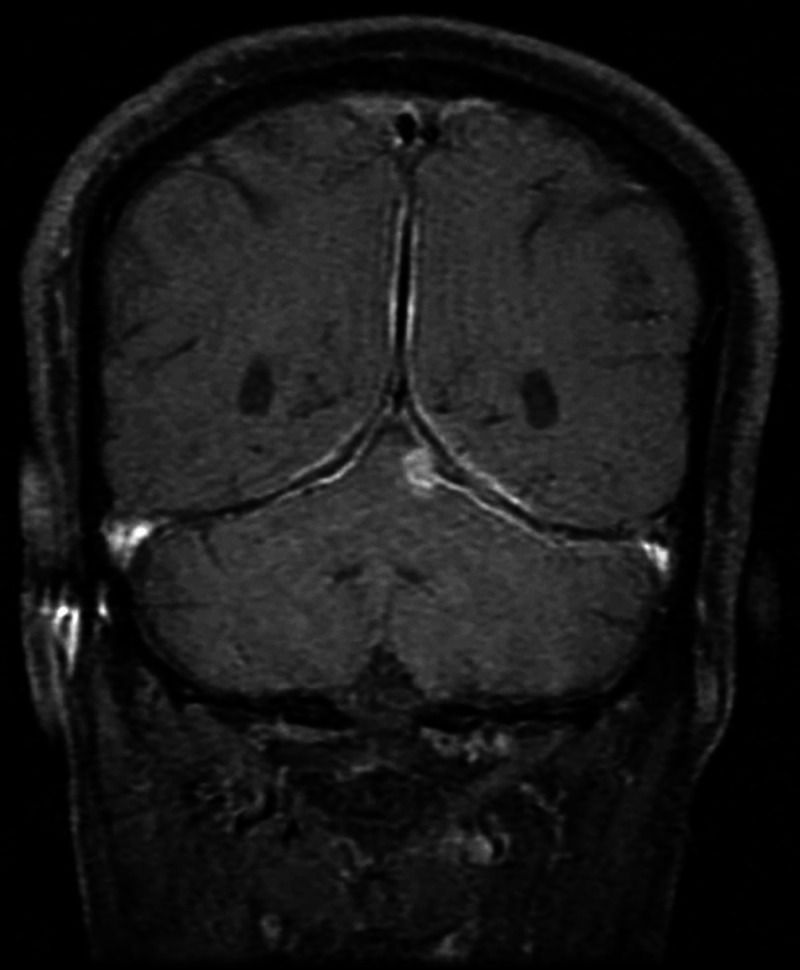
Posttreatment at 24 months: Post-gadolinium coronal T1-weighted MRI showing almost complete resolution of dural enhancement. MRI: magnetic resonance imaging
